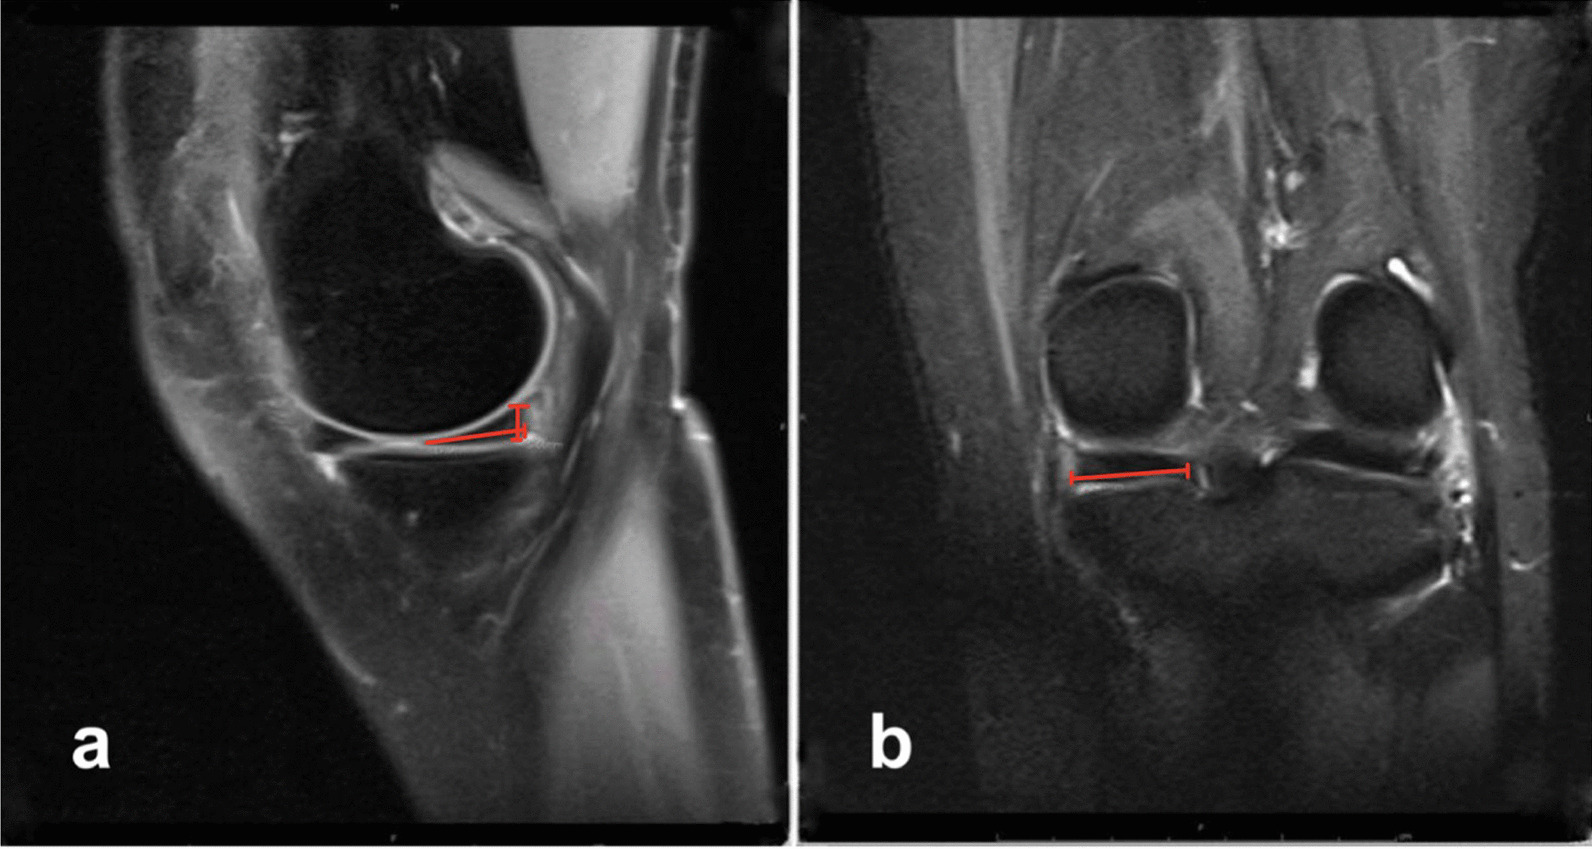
The measurement of medial meniscus posterior horn a height, a length, and b depth on a proton density sagittal magnetic resonance image
